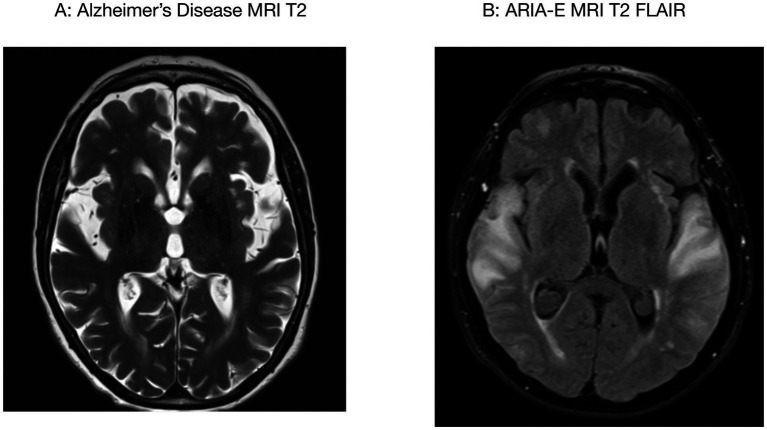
(A) Alzheimer’s disease MRI T2. (B) ARIA-E MRI T2 FLAIR.
